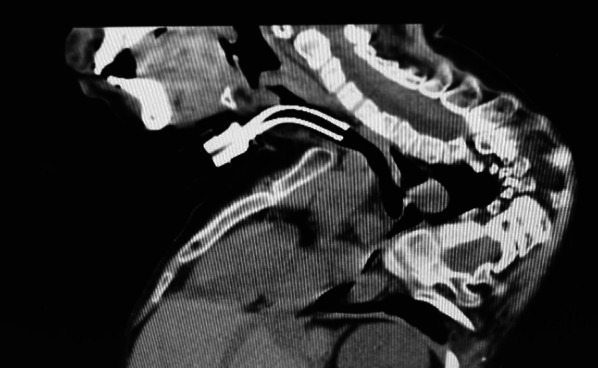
Computed tomography (CT) image at 1 month after tracheostomy. CT shows no ossification at the surgical site or abnormalities in cannula placement
